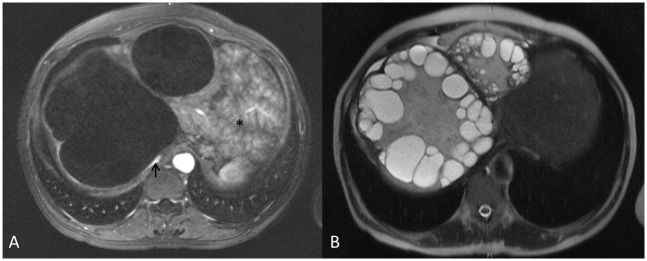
A: contrast enhanced MRI with compression of caval vein (arrow) and inhomogeneous perfusion of liver tissue (pseudo-Budd-Chiari syndrome, asterisk); B: T2-weighted MRI displaying solid and liquid components of CE 3b cysts.
